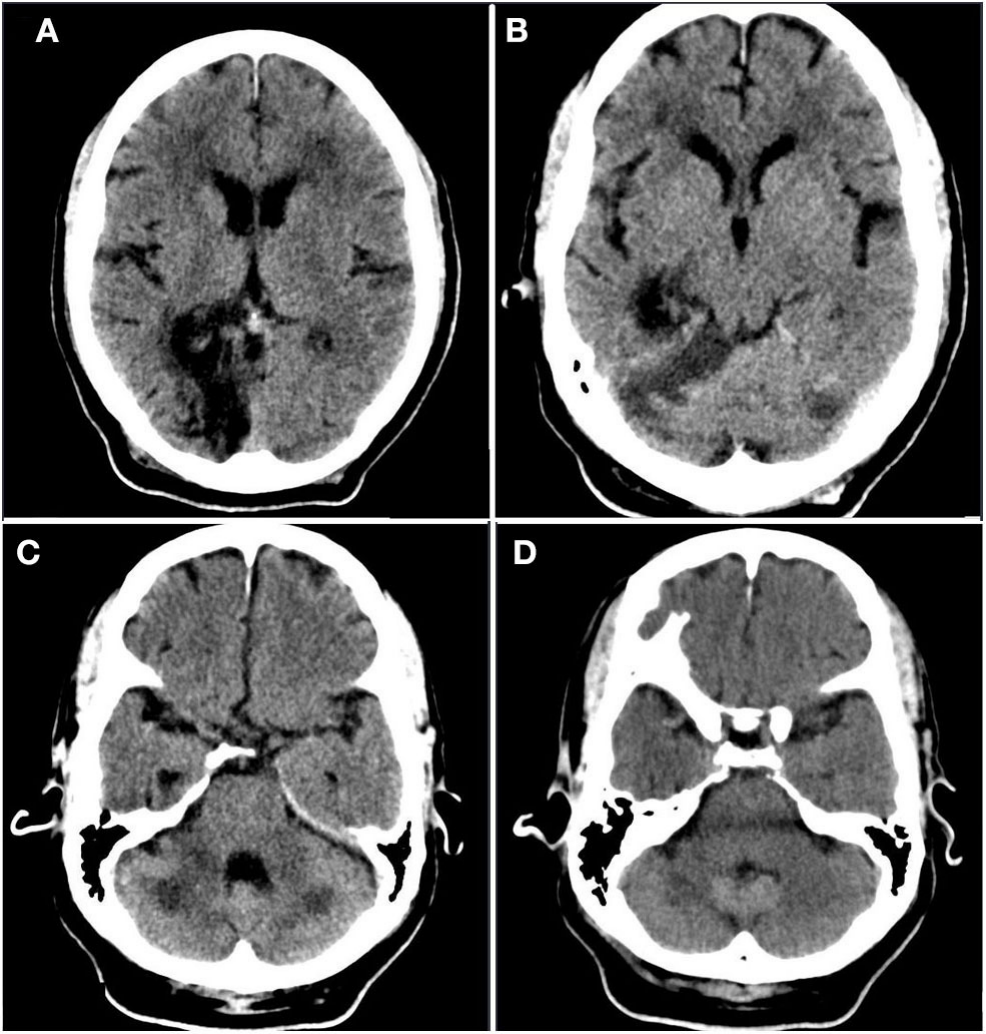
Prior infarct. Rostral to caudal (A–D) non-contrast computed tomographic axial slices of the head performed 2 years prior to current admission demonstrates hypoattenuation and parenchymal volume loss in the right parasagittal occipital lobe compatible with an old posterior cerebral artery territory infarct, as well as acute infarct in the territory of the bilateral superior cerebellar arteries.
